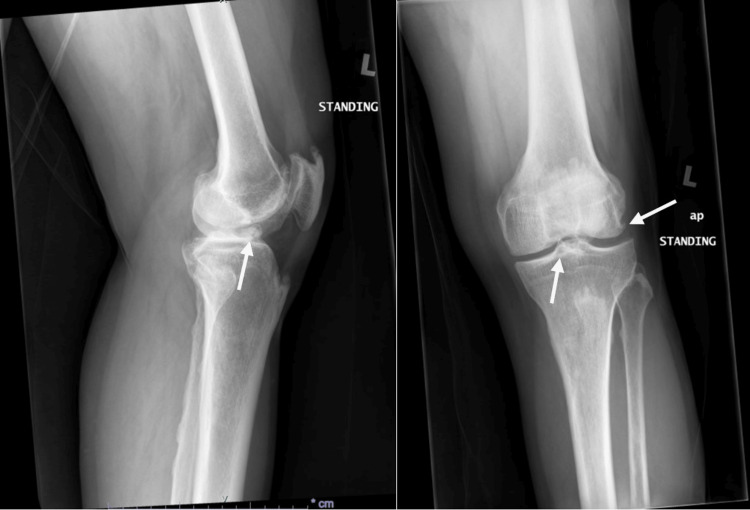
Plain radiograph showing marginal osteophytes and presence of radiopaque body over the left knee
